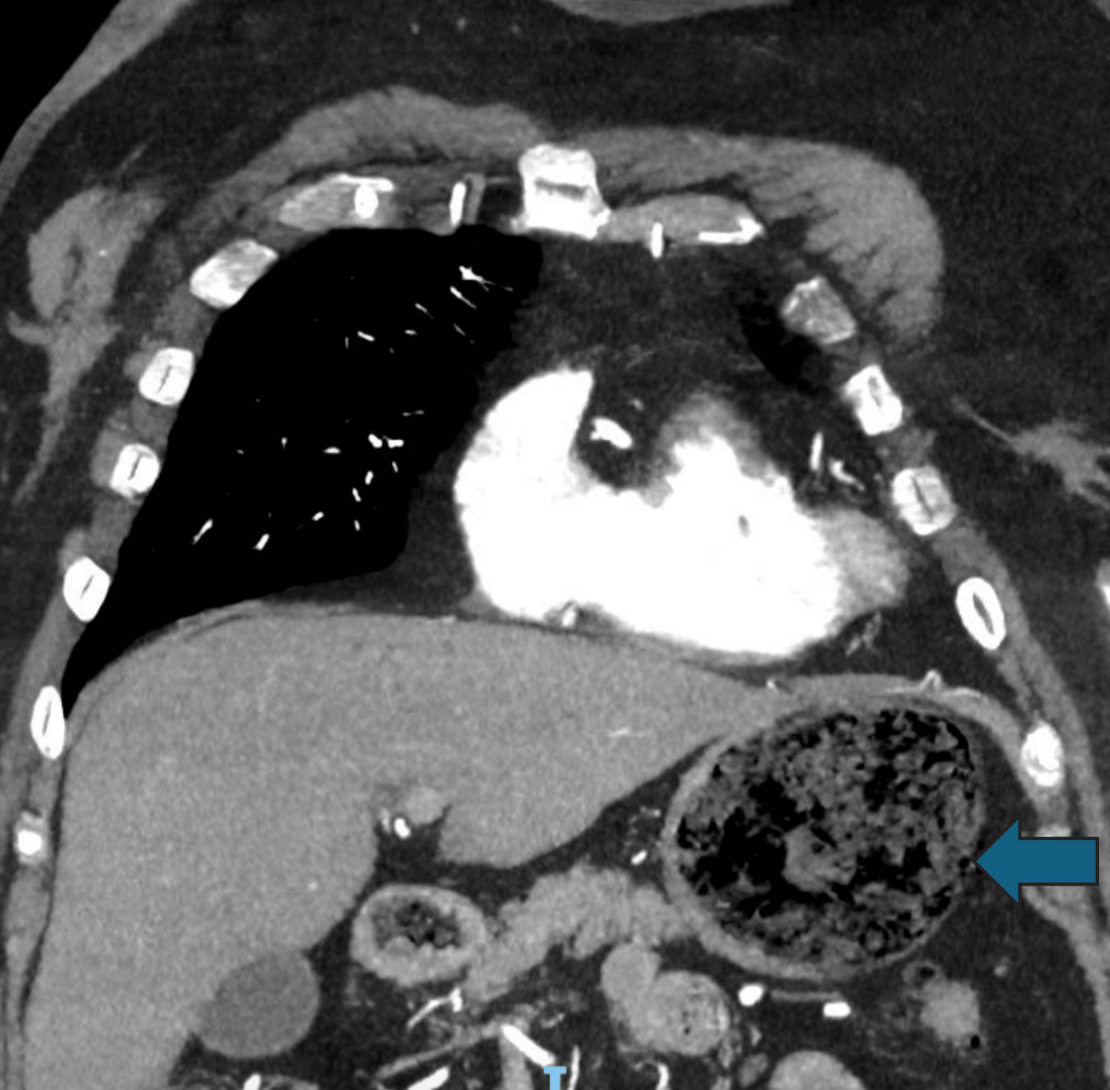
CT angiography showing mild intramural gas within the stomach (arrow), suggestive of emphysematous gastritis
